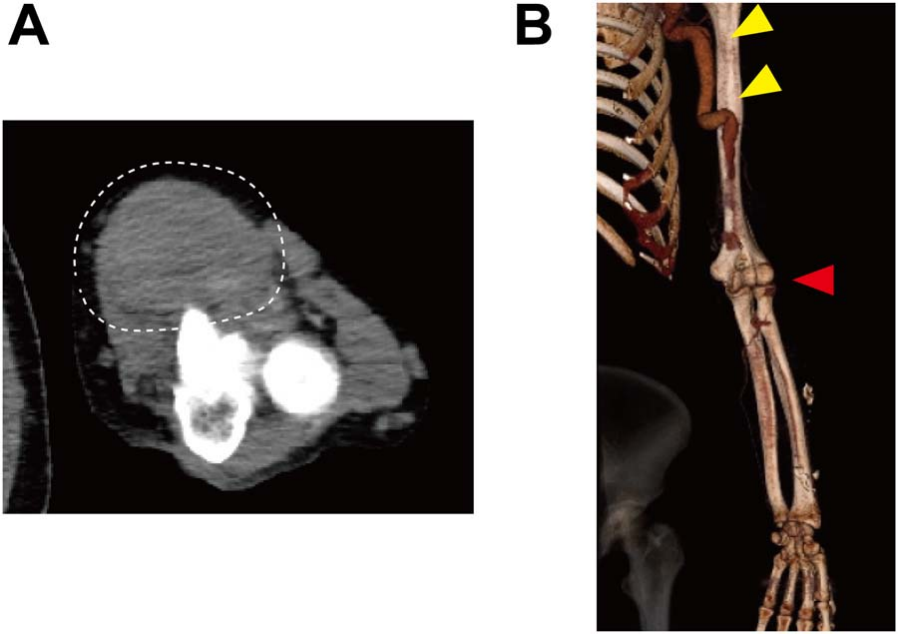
Preoperative contrast CT showing (A) a true brachial aneurysm at the anterior aspect of the elbow joint, which was obstructed by a thrombus (white dashed circle), and (B) the dilated brachial artery (yellow arrows). Red arrow indicates the level of the true brachial aneurysm.
